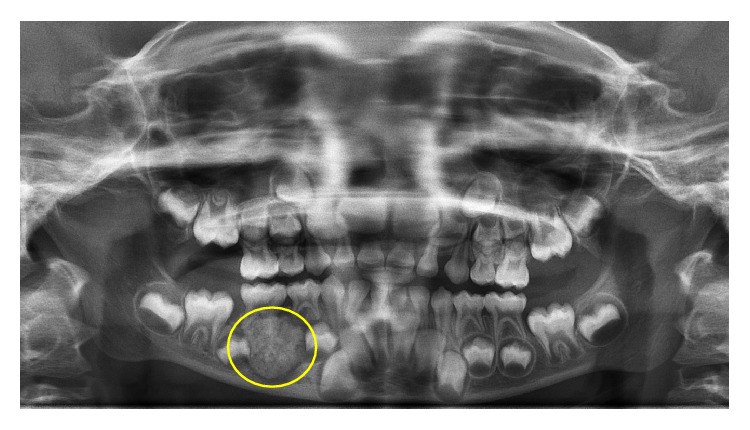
Preoperative panoramic radiograph with radiopacity surrounded by a radiolucent border associated with mesial root of lower right second primary molar.
